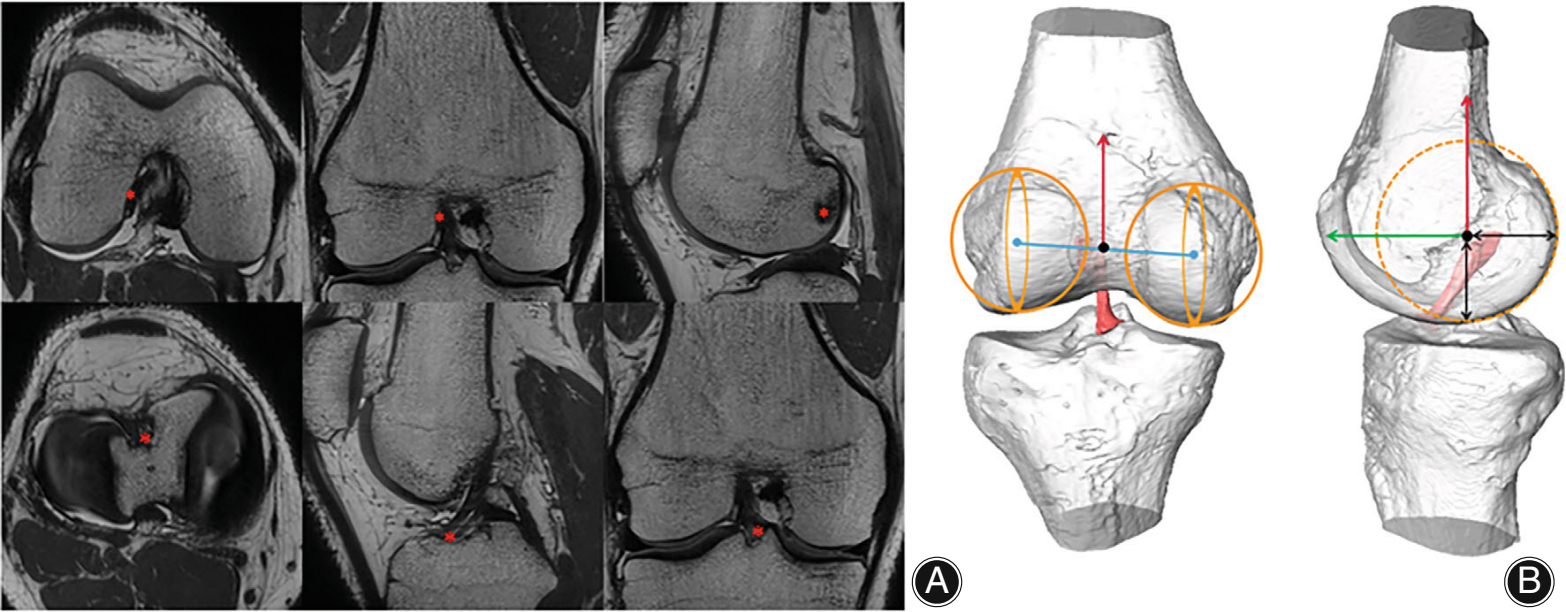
(A) Proton density 3D fast spin‐echo volume sequences (PD space) were applied to collect high‐resolution volumetric MR images, the ACL footprint position is marked with an asterisk. (B) Three‐dimensional surface models of the femur, tibia and ACL were reconstructed. The anatomic coordinate system of the femur is constructed. The origin is shown with a black circle; the mediolateral axis, connecting the center of the best‐fitted spheres on the posterior articular surface of the medial and lateral condyles, is shown with a blue line. The distal‐proximal is demonstrated with a red arrow and the anterior‐posterior axis, a green arrow. The lateral femoral condyle width, defined as the radius of the best‐fitted sphere in the lateral condyle, is shown with a black arrow. ACL, anterior cruciate ligament; MR, magnetic resonance.
